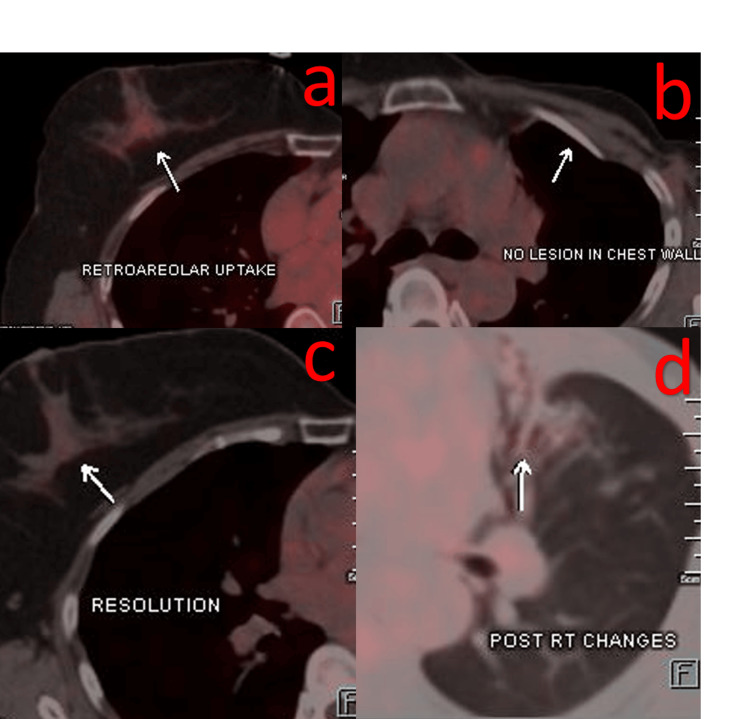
PET-CT scan report post 12 cycles of T-DM1 in March 2022 suggestive of resolution of previously seen retro areolar uptake in the right breast and no lesion in the chest wall (a, b). Resolution of liver deposits and post-RT changes (c, d).
